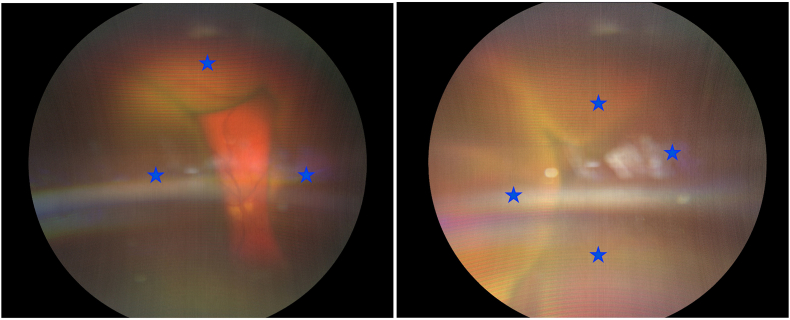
B-mode ultrasound confirmed the presence of a severe choroidal detachment with kissing sign without a visualized intraocular mass (Fig. 2: B-mode ultrasound confirmed the presence of a severe bilateral choroidal detachment with kissing sign, arrow: voluminous choroidal detachment).
